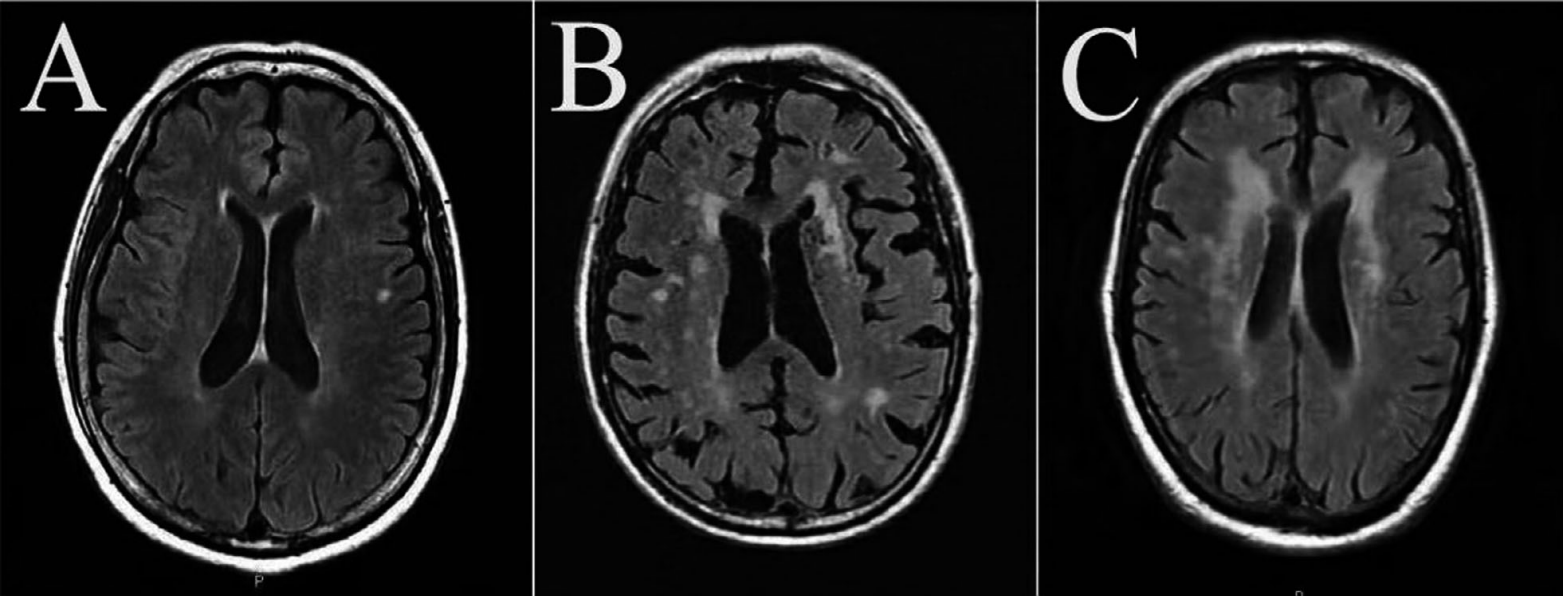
Representative T2‐FLAIR images of WMH A: PVH grade 1 and DWMH grade 1; B: PVH grade 2 and DWMH grade 2; C: PVH grade 3 and DWMH grade 3. Abbreviations: WMH, white matter hyperintensities; PVH, periventricular hyperintensities; DWMH, deep subcortical white matter hyperintensities.
